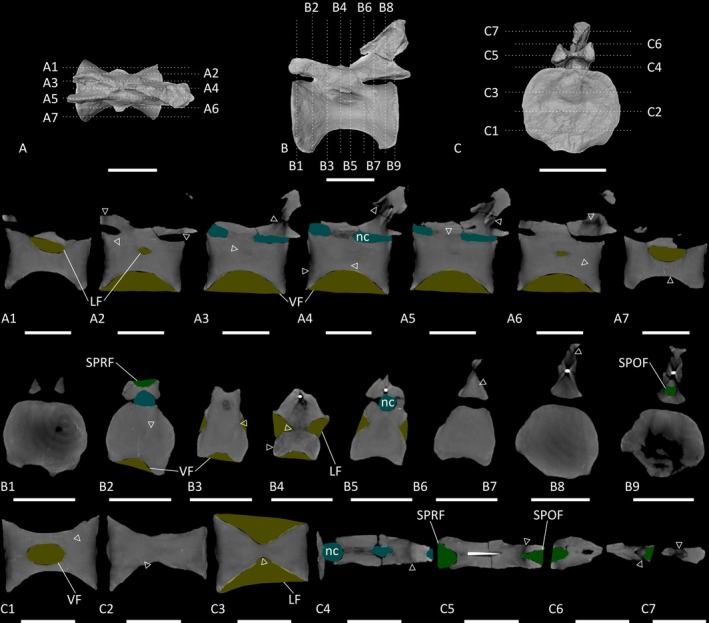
CT scan of *Tornieria africana* posterior caudal vertebra MB.R.2956.14. (A–C) 3D rendering (A) dorsal view, (B) left lateral view, (C) anterior view; (A1–A7) CT scan slices in parasagittal cross‐section, (B1–B9) CT scan slices in transverse cross‐section, (C1–C7) CT scan slices in horizontal cross‐section. LF, lateral fossa; nc, neural canal; SPOF, spinopostzygapophyseal fossa; SPRF, spinoprezygapophyseal fossa; VF, ventral fossa. Key: Blue highlight, neural canal; green highlight, neural spine fossa; yellow highlight, centrum fossa; white arrow, pneumatic bone texture. Scale bars = 100 mm.
